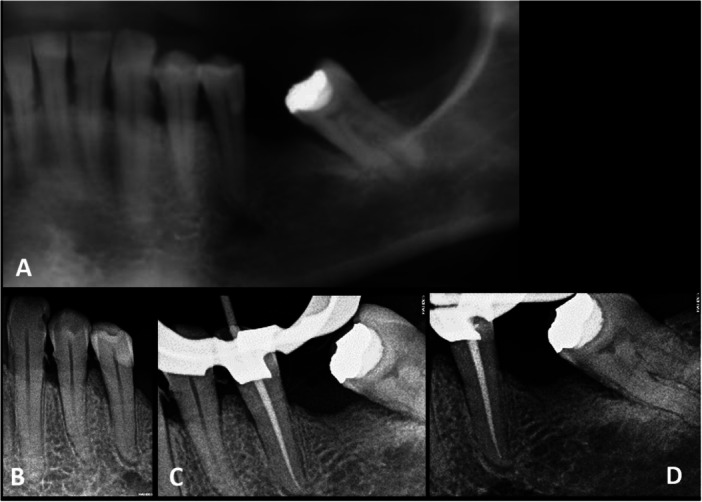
Initial radiological results of the lower left second premolar. (A) Panoramic X‐ray focused on the lower left second premolar, indicating a periapical index score of 2 for this tooth, presenting clinical signs of acute periapical periodontitis and normal response to pulpal tests. (B) Preoperative X‐ray showing a PAI score of 2. (C) Per operative X‐ray with gutta‐percha master cone. (D) Postoperative X‐ray.
